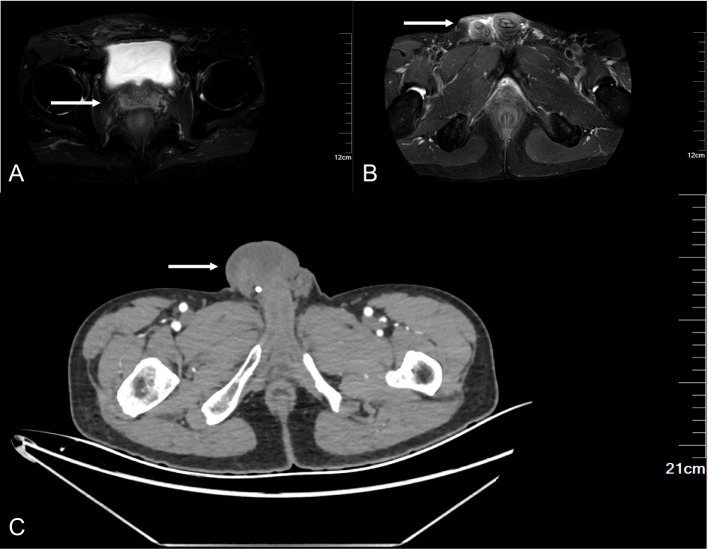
Imaging examination. (A, B) MRI (C) Enhanced CT.
